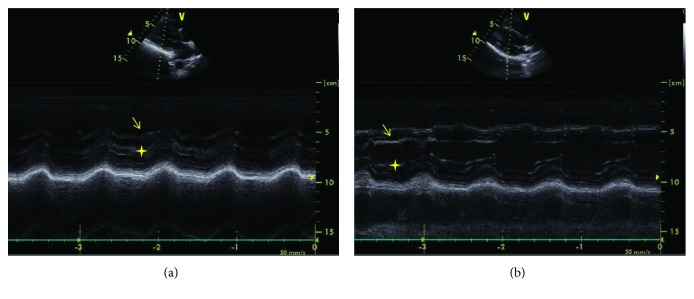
M-mode echocardiographic image from the father (a) and his daughter (b). (a) and (b) M-mode recording from the midsegment of the left ventricle. The arrow indicates the false tendon; The star indicates the papillary muscles.
